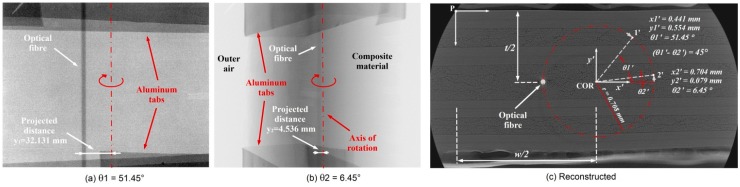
(a,b) Two X-ray projections taken at different angles (*i.e.*, −45° and 0°) clockwise with respect to the centre of rotation. The position of the OF is shifting from left to right due to its eccentricity with respect to the rotational axis of the manipulator. The darker regions above and below each projections are aluminium tape stripes attached to the sample; (c) Coordinates of the OF for the two different orientations were obtained using the formulas.
